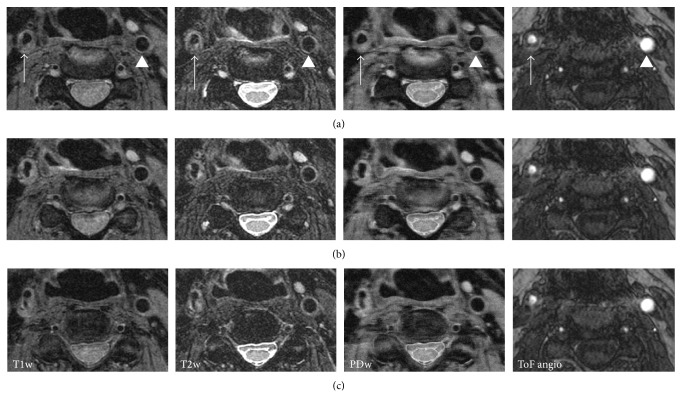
Example of multisequence MRI of a plaque in the right carotid artery (arrow). Each column shows three slices from the right common carotid artery (top row) to the internal carotid artery (bottom row). The nonstenotic left coronary artery is shown for comparison (arrowhead). Four different MR sequences are shown (T1 weighted, T2 weighted, proton density weighting, and time-of-flight angiography).
